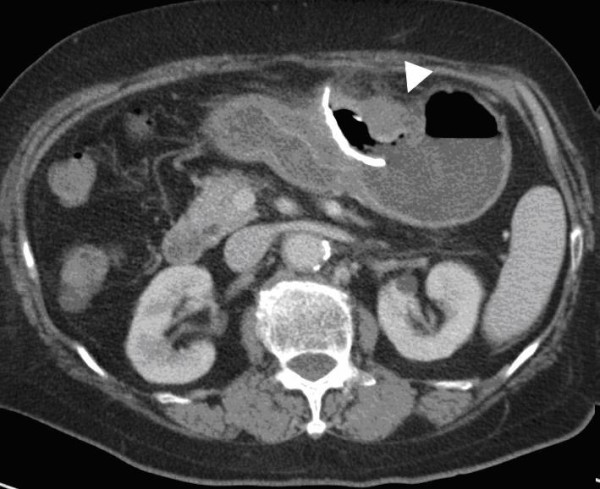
Pre-operative CT scan showed gastric mass with curvilinear calcification penetrating into surrounding soft tissue. Localized abscess formation was noted. A gastric tumor adjacent to the calcification was noted.
